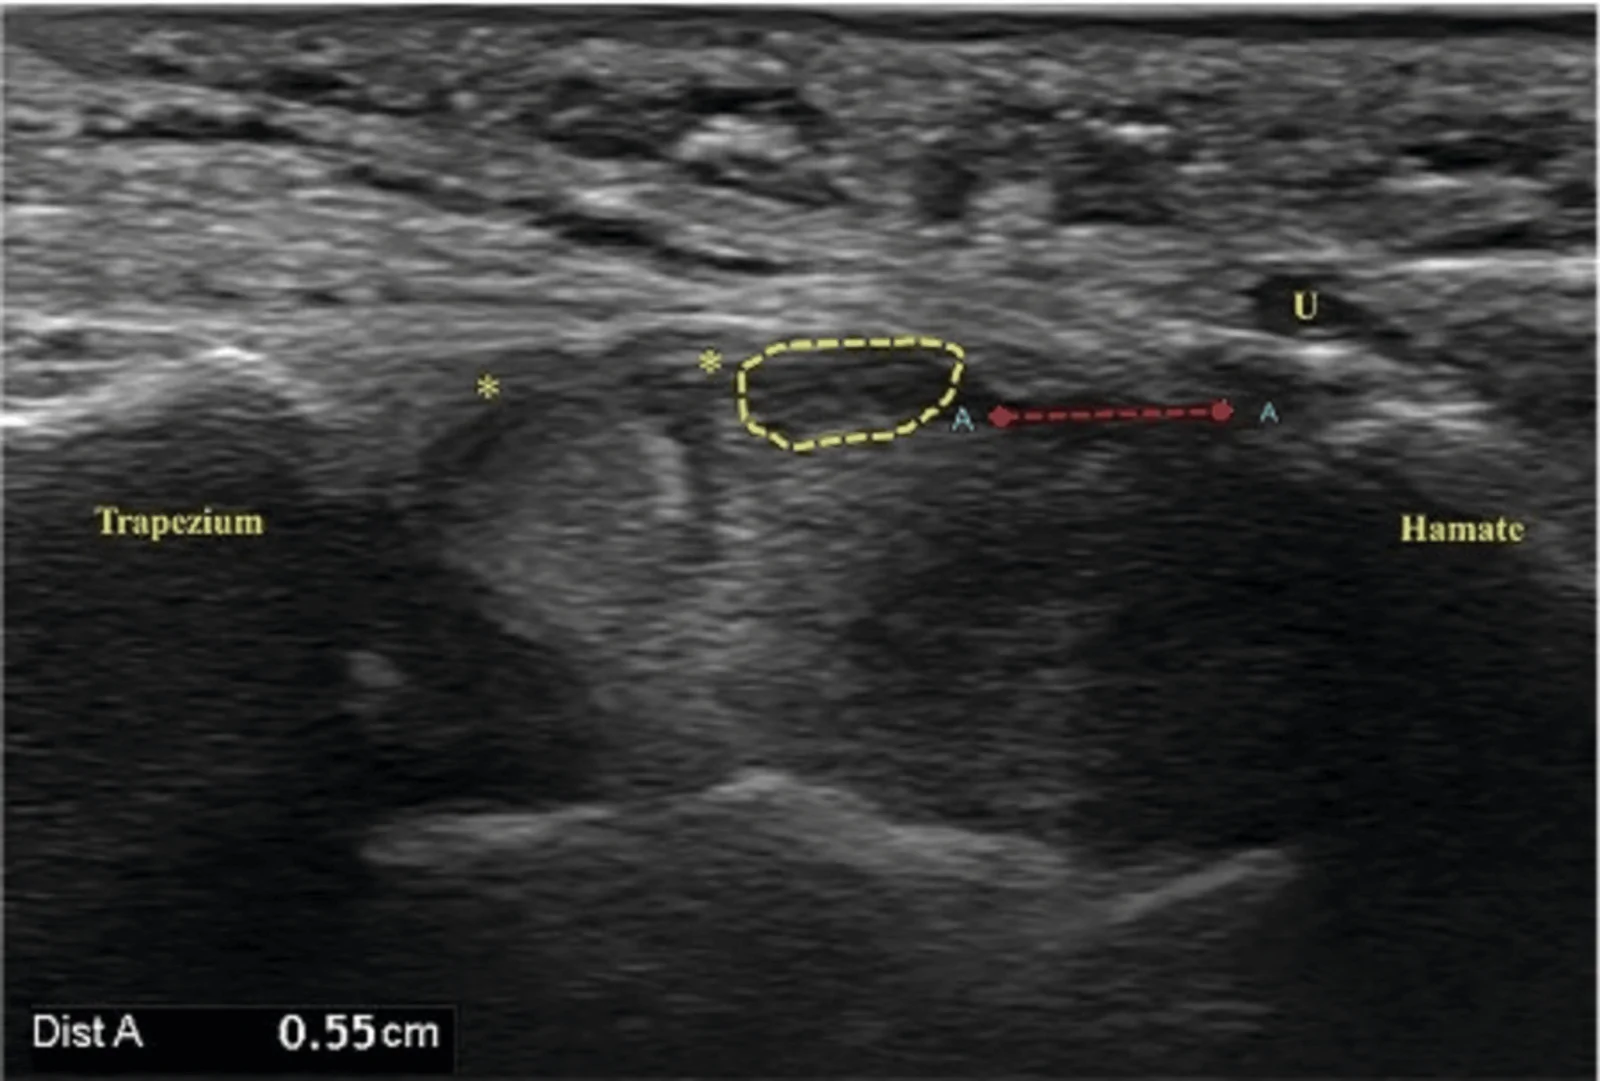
Ultrasonographic assessment of median nerve mobility at the carpal tunnel inlet. The yellow dashed outline indicates the median nerve, and the red dotted line represents the measured distance from the pisiform bone to the median nerve with the wrist in a neutral position.
